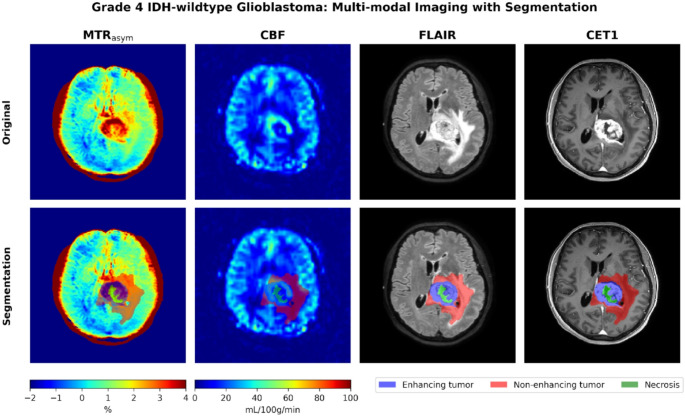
Magnetization transfer ratio asymmetry (MTR_asym_), cerebral blood flow (CBF), Fluid-Attenuated Inversion Recovery (FLAIR), and post-contrast T1 (CET1) scans of a grade 4 IDH-wildtype glioma case with segmentation labels for enhancing tumor (blue), non-enhancing tumor (red) and necrosis (green)
